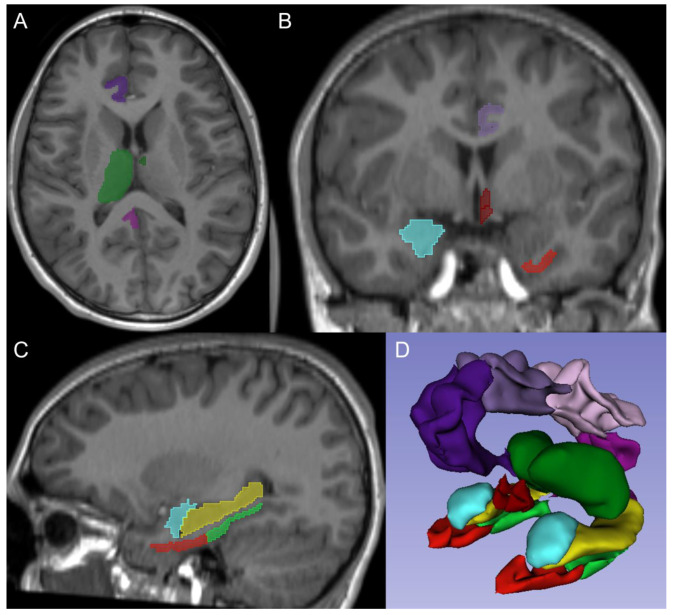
Regions of interest overlay. (A–C) demonstrate axial, coronal, and sagittal T1-weighted MR images with right cingulate gyrus (pink and purple), whole right thalamus (green), and left antero-ventral nucleus of the thalamus (dark green) in (A); right amygdala (light blue), left hypothalamus (dark red), left cingulate gyrus (purple), and left entorhinal cortex (light red) in (B); and left amygdala (light blue), left entorhinal cortex (dark red), left parahippocampal cortex (green), and left hippocampus (yellow) in (C). (D) shows a 3D reformat of the regions of interest using the same color coding as (A–C).
